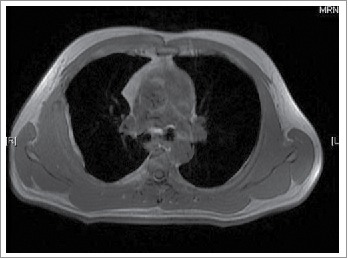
MRI demonstrates thickened soft tissue lesion four months after surgery.
